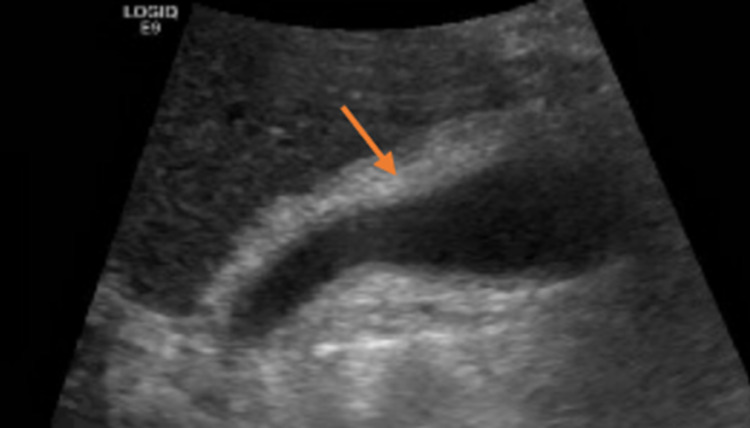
RUQ US demonstrating mild GB wall thickening (arrow) without evidence of gallstones RUQ - right upper quadrant; US - ultrasound; GB - gallbladder
